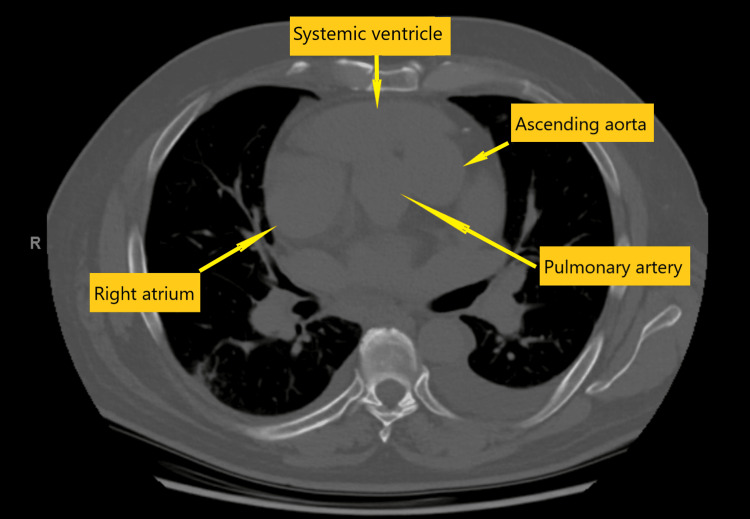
Preoperative CT scan The ascending aorta, arising from the morphological right ventricle (systemic ventricle), is positioned to the left of the pulmonary artery.
